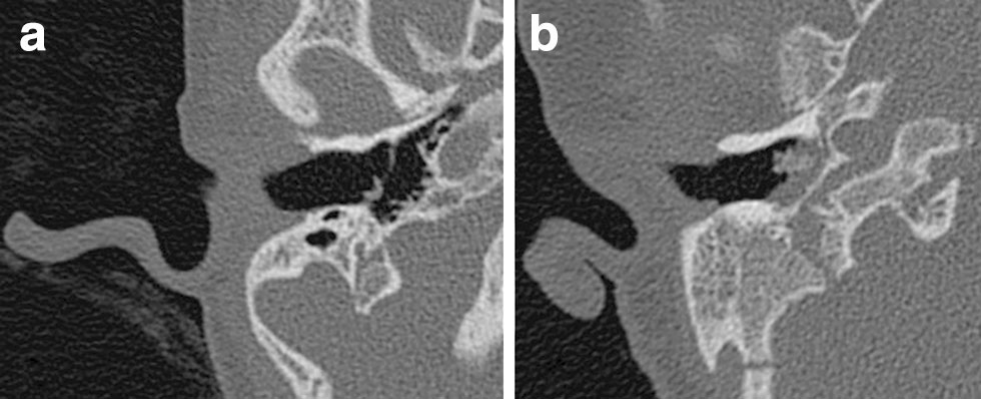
Mastoid size—axial CT images of a small mastoid (a) and a wide mastoid (b); both ears had a grommet in situ
